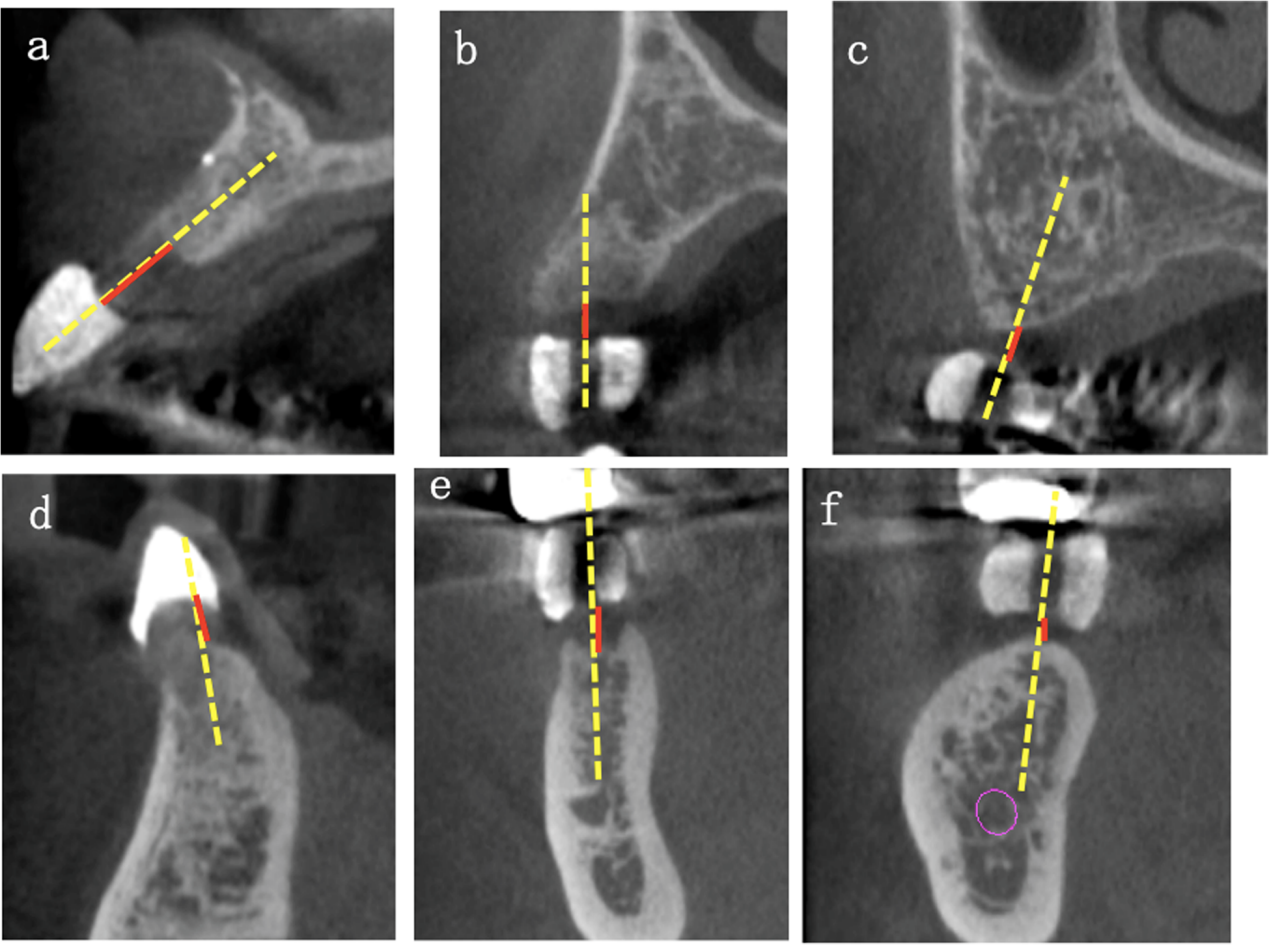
Cross-sectional image. The yellow dotted line indicates the ideal direction of implant insertion, as determined based on the radiographic stent, and set as the long axis. Red lines indicate the mucosal thickness as measured from the base of artificial teeth of the diagnostic template to the surface of the alveolar bone. a Maxillary anterior region. b Maxillary premolar region. c Maxillary molar region. d Mandibular anterior region. e Mandibular premolar region. f Mandibular molar region
